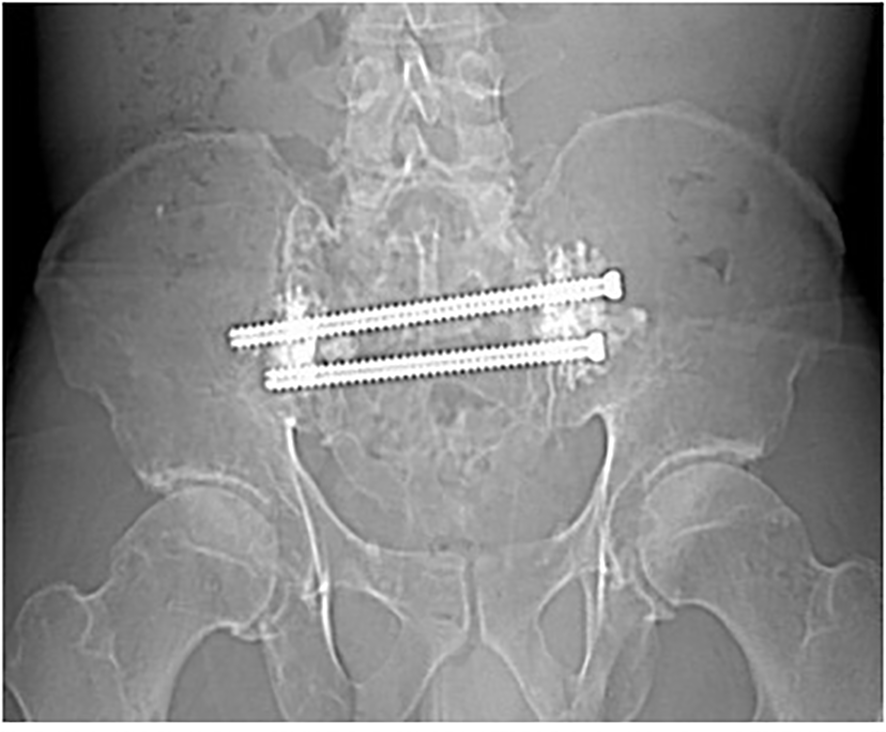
Radiograph made 9 months following CT-Fluoroscopic guided trans-sacral screw fixation with sacroplasty, showing stability with no evidence of screw loosening.
